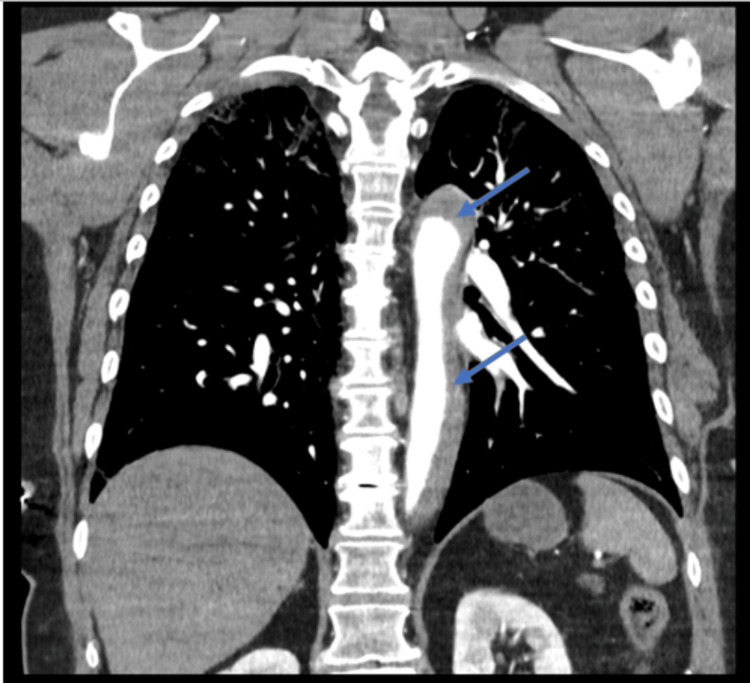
CTA of the chest (coronal view) with and without IV contrast showing acute type A Stanford dissection (arrows) involving the ascending aorta with significant extension into the descending thoracic aorta CTA: CT angiography
